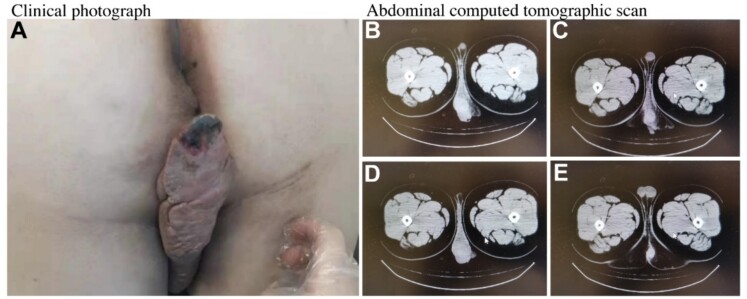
The soft mass and computed tomographic scan of the pelvis.
